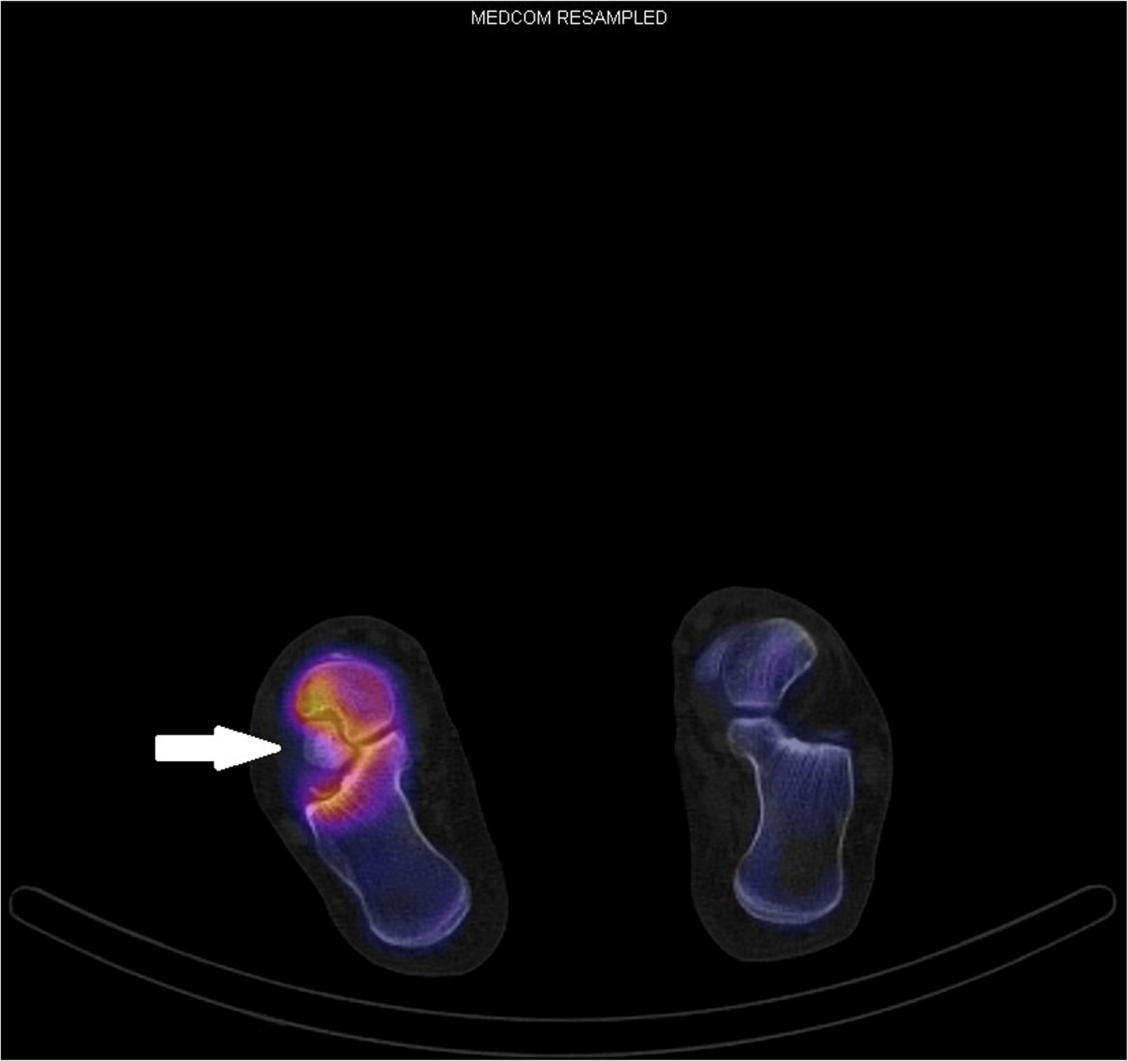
Preoperative single-photon emission computed tomography-computed tomography scan, transversal plane. Activity around the calcaneus secundarius (arrow) visible.
